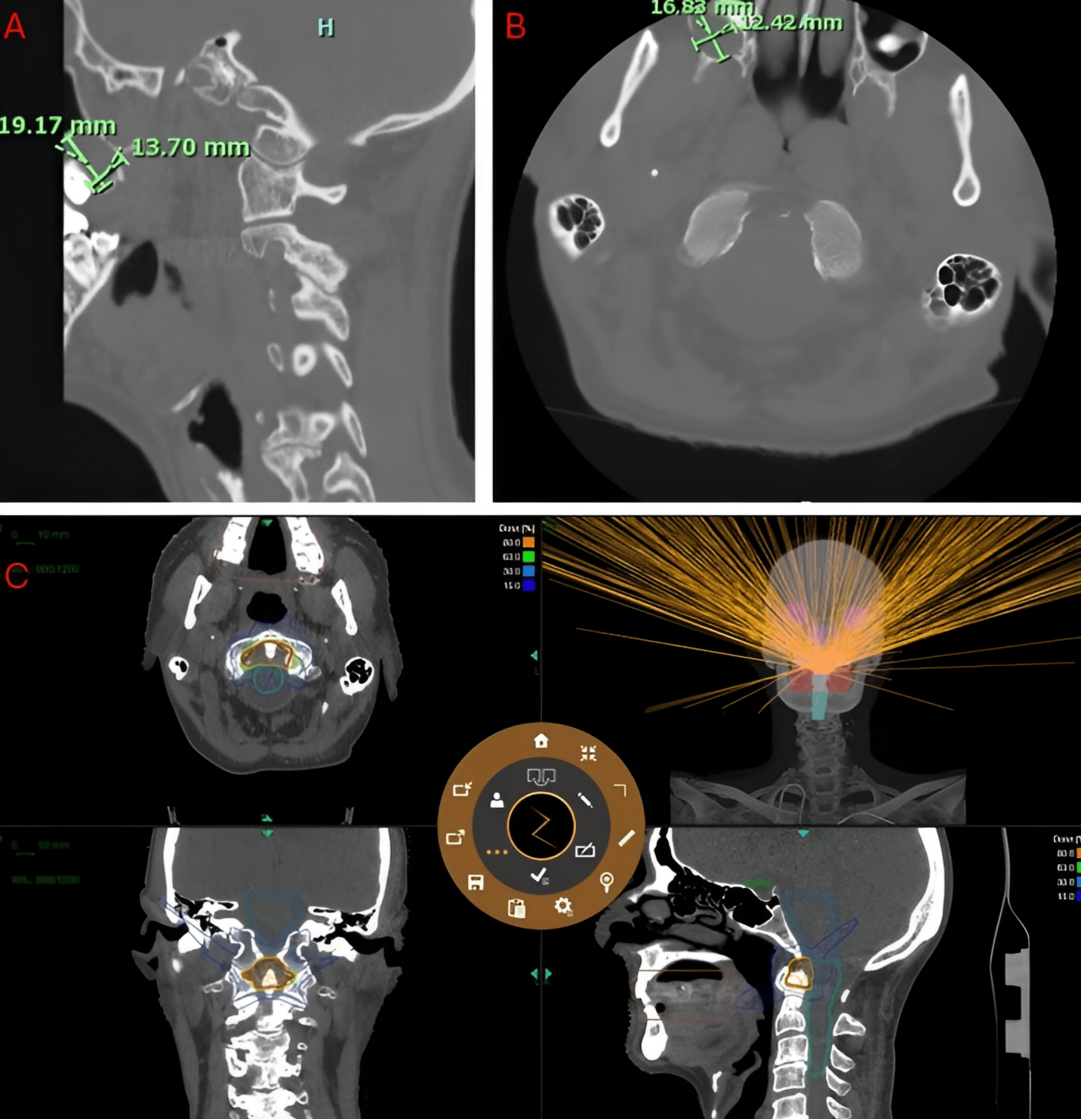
ZAP-X treatment planning images of Patient 3. A,B: CT scan (bone window) showing metastasis to the C2 vertebral body with marrow replacement; C: ZAP-X TPS contours and dose delivery visualizations.
